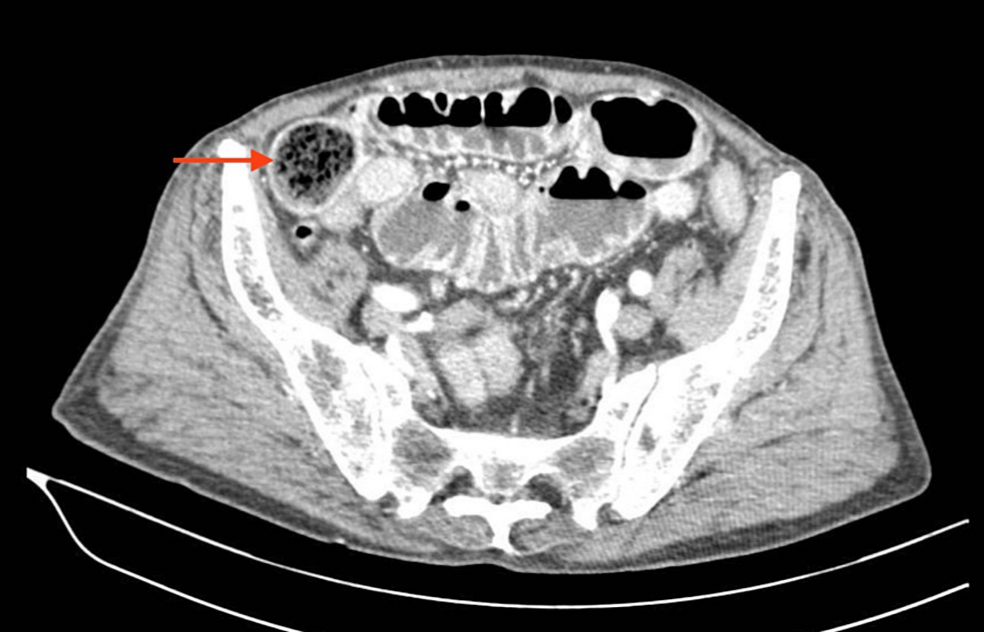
Axial contrast-enhanced abdominal CT scan revealing an oval mottled-appearing mass suggesting a bezoar (arrow), with proximal small bowel distension.
